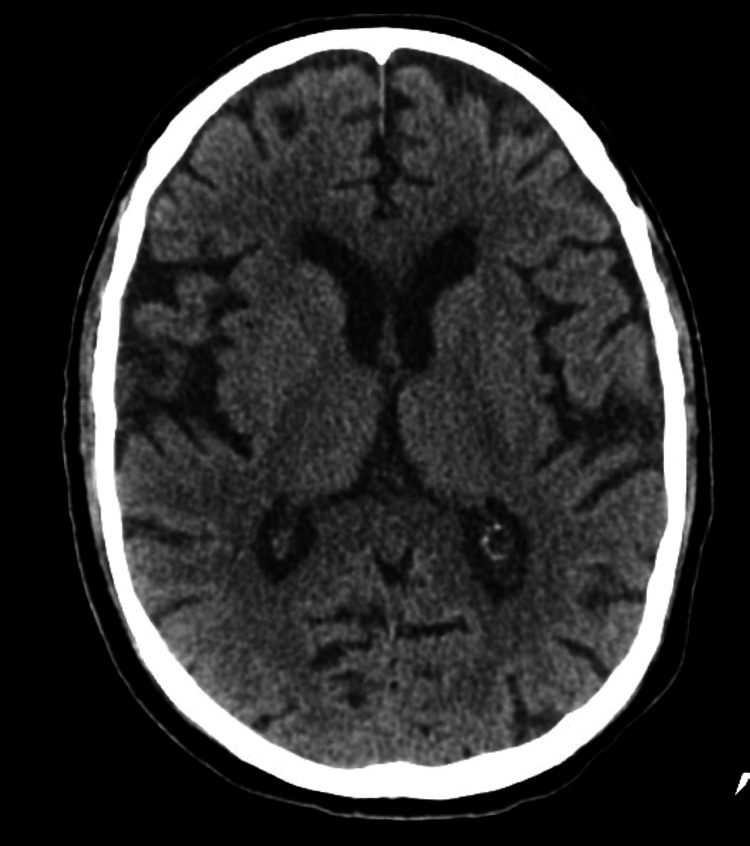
CT scan of the head showing no gross abnormality
